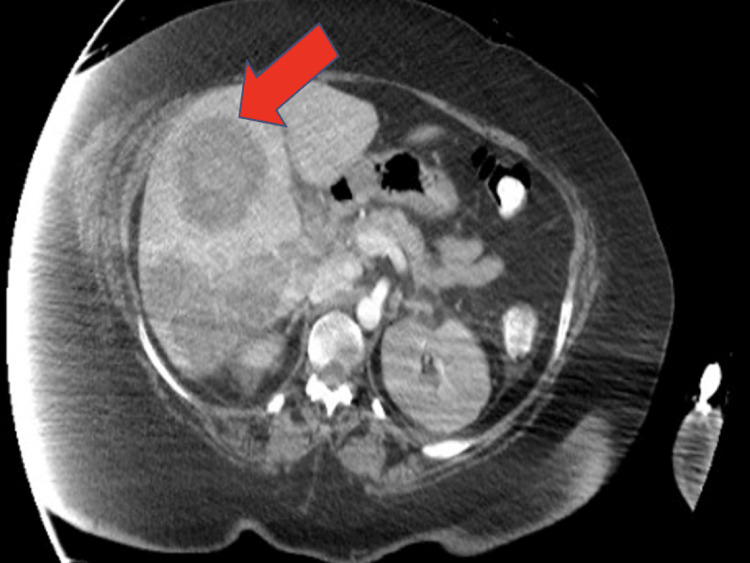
CT abdomen on day 19 showing multiple hyperdense liver lesions. The red arrow points to the largest collection. CT: computed tomography
